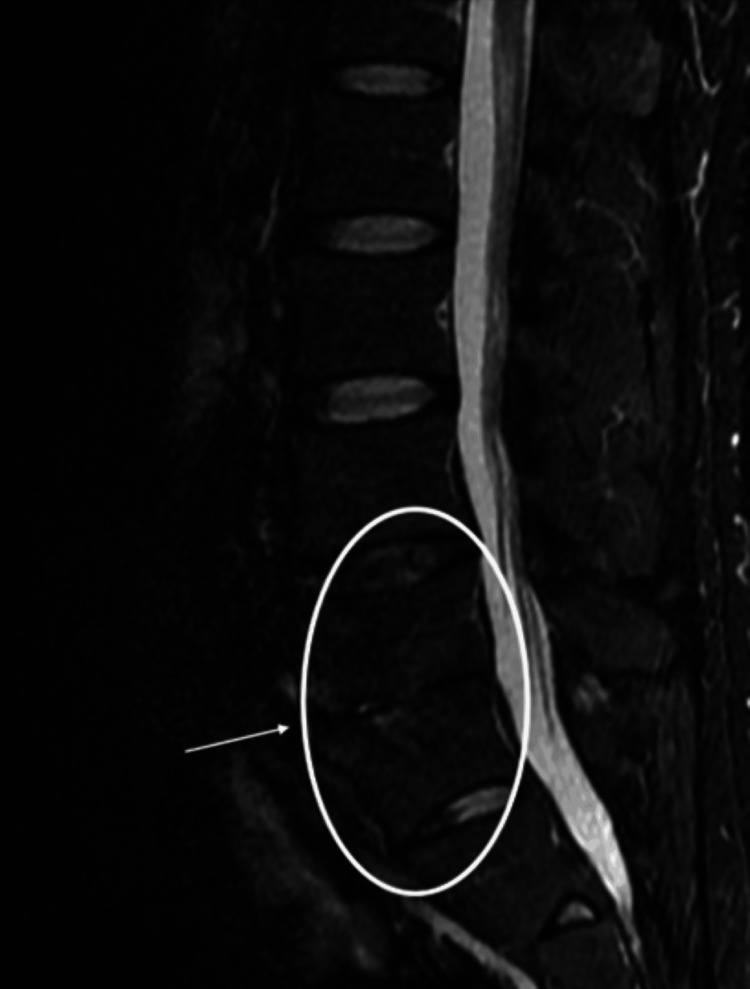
Post-treatment lumbar MRI Follow-up lumbar spine MRI after antimicrobial therapy for pyogenic spondylitis. Compared to the initial study, STIR images show a marked decrease in high signal intensity at the L4–S1 intervertebral spaces (the circled area in the image), indicating resolution of bone marrow edema. T1-weighted images demonstrate partial normalization of signal intensity in the L4 vertebral body, and contrast-enhanced fat-suppressed T1-weighted images show reduced enhancement at the L4–S1 endplates and adjacent soft tissue, consistent with decreased inflammatory activity. MRI: magnetic resonance imaging, STIR: short tau inversion recovery
